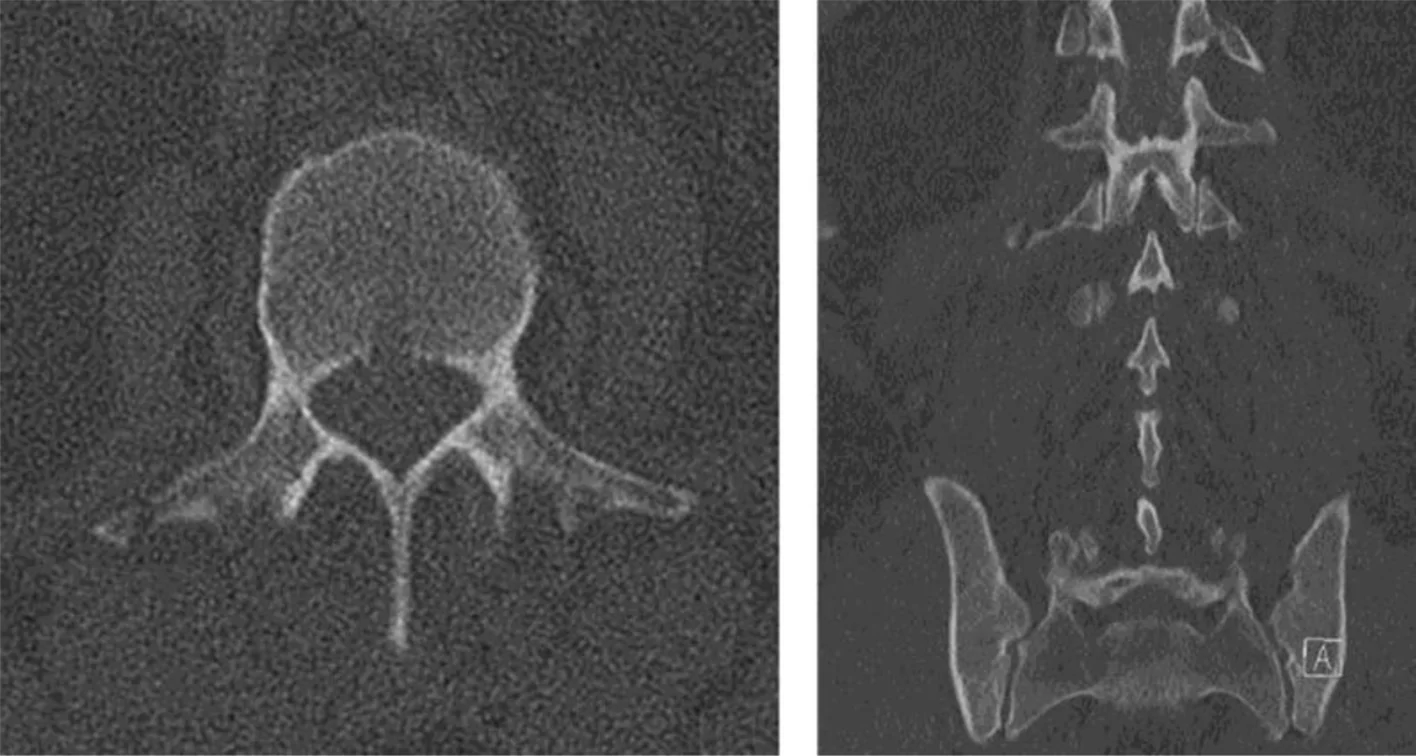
Axial and coronal CT scans of an isolated lumbar transverse process fracture [56]; reproduced from Peterson et al. [56], with permission
